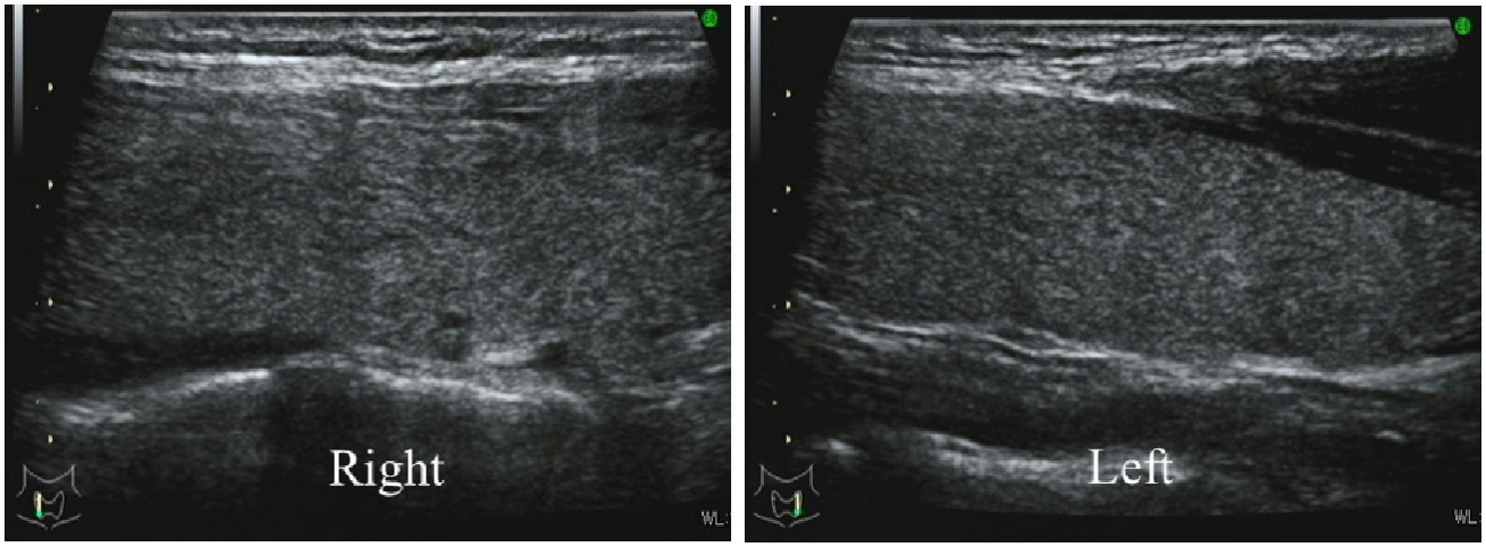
Sagittal ultrasonography of the thyroid gland at 1 month postpartum in case 1.
